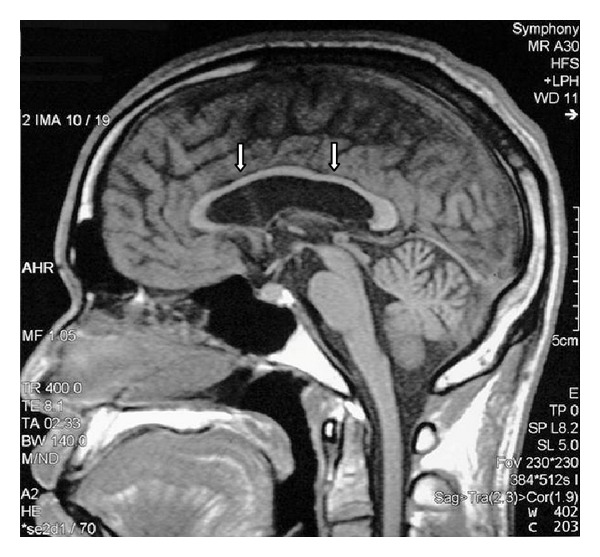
Sagittal T1 weighted MR image of the brain shows thinning of the corpus callosum (arrows). Cerebellar atrophy also evident with enlargement of cerebellar sulci.
